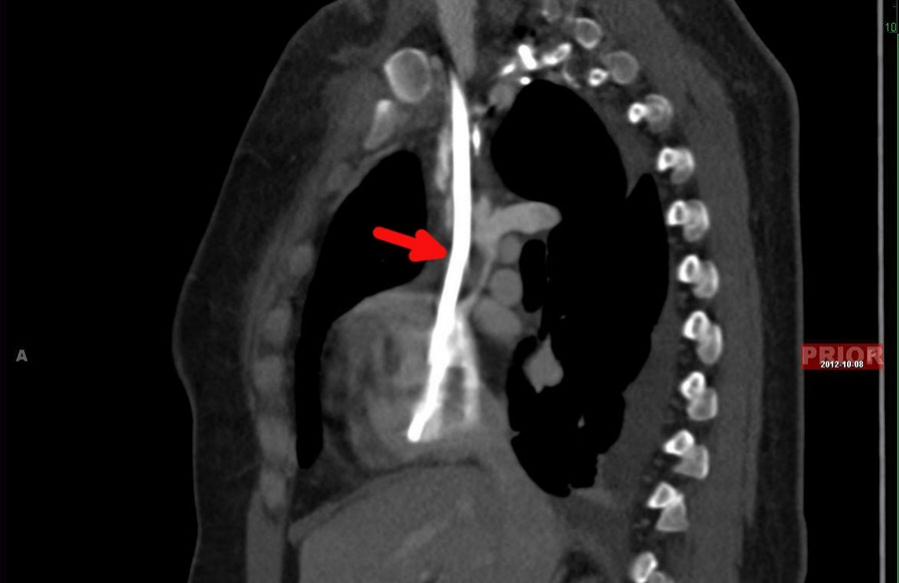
CT chest with contrast (*sagittal view*) demonstrating catheter in the SVC (*bright white* shown by *red arrow*) with surrounding thrombus
